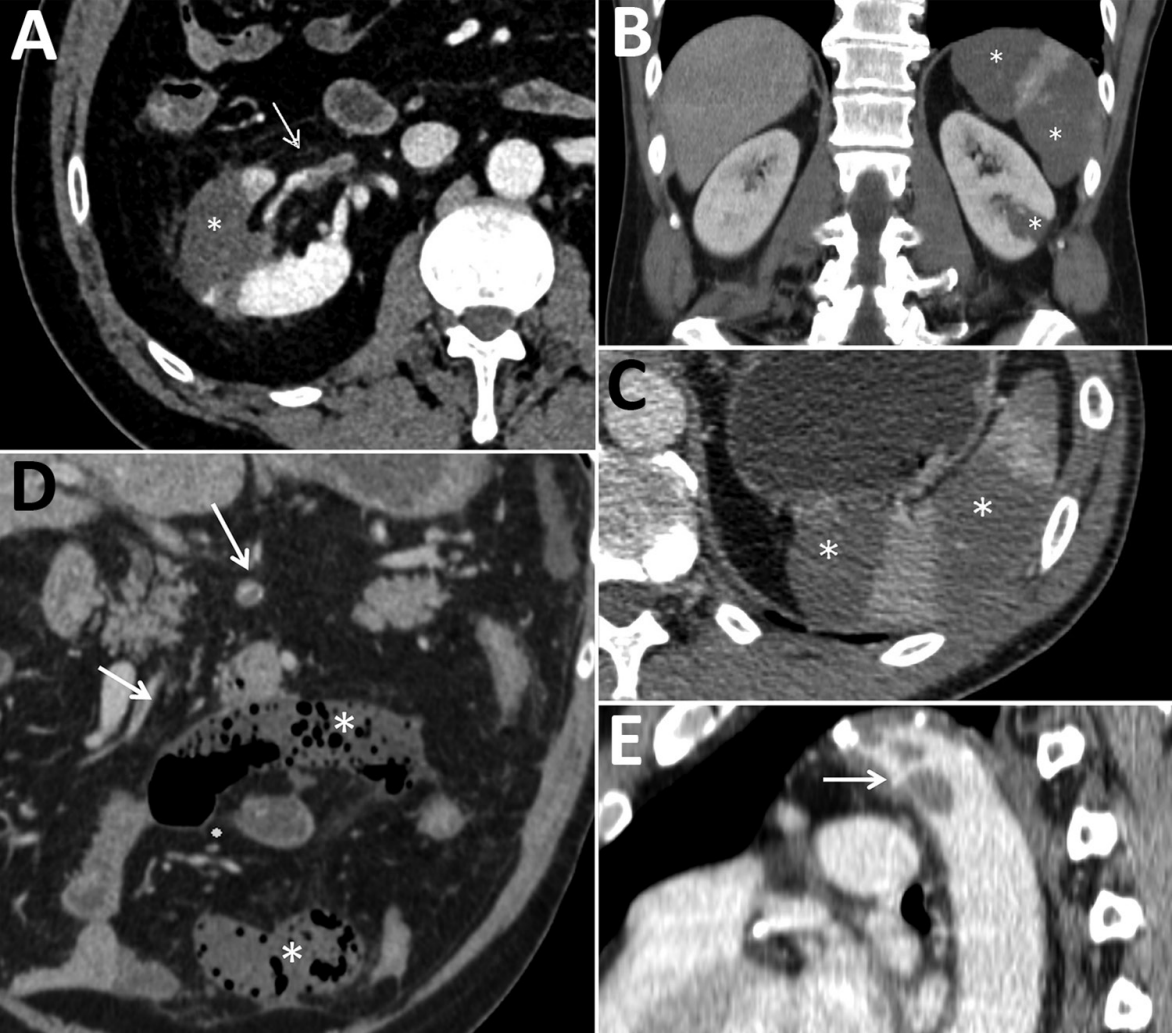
Abdominal contrast-enhanced computed tomography scans of 3 coronavirus disease patients with abdominal visceral infarction, Italy. A) Patient 1 (axial view) showing intraarterial thrombi in the renal artery (arrow) and kidney and splenic infarctions (asterisk), seen as large wedge-shaped hypodense parenchymal areas. B, C) Patient 2 (B, coronal view; C, axial view) showing kidney and splenic infarctions (asterisks), seen as large wedge-shaped hypodense parenchymal areas. D, E) Patient 3 (D, coronal view; E, sagittal view), showing intraarterial thrombi in the superior mesenteric artery and its branches (arrows in D) and thoracic descending aorta (arrow in E), as well as small bowel ischemia (asterisks in D), seen as small bowel loops with decreased or absent wall enhancement. In patients 1 and 2, scans did not show notable signs of atherosclerosis.
